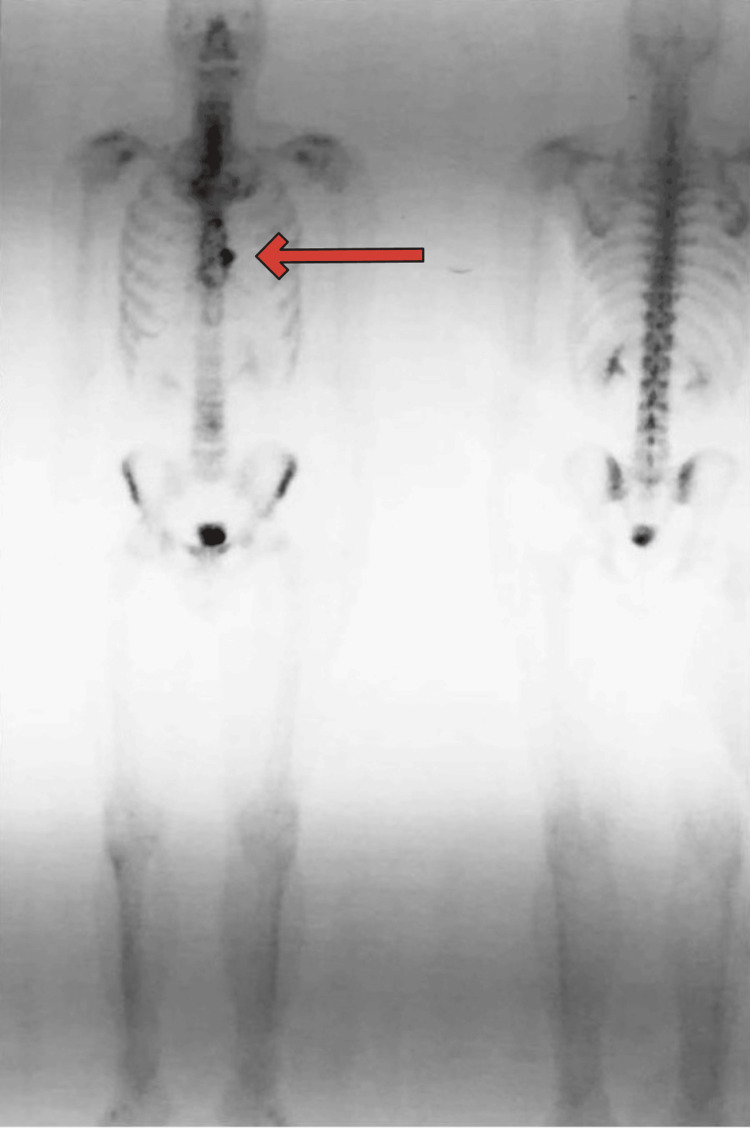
Scintigraphy showing hyperfixation of the radiopharmaceutical in the body of the sternum Bone scintigraphy using Technetium-99m shows pathological hyperfixation in the body of the sternum, as indicated by a red arrow.
